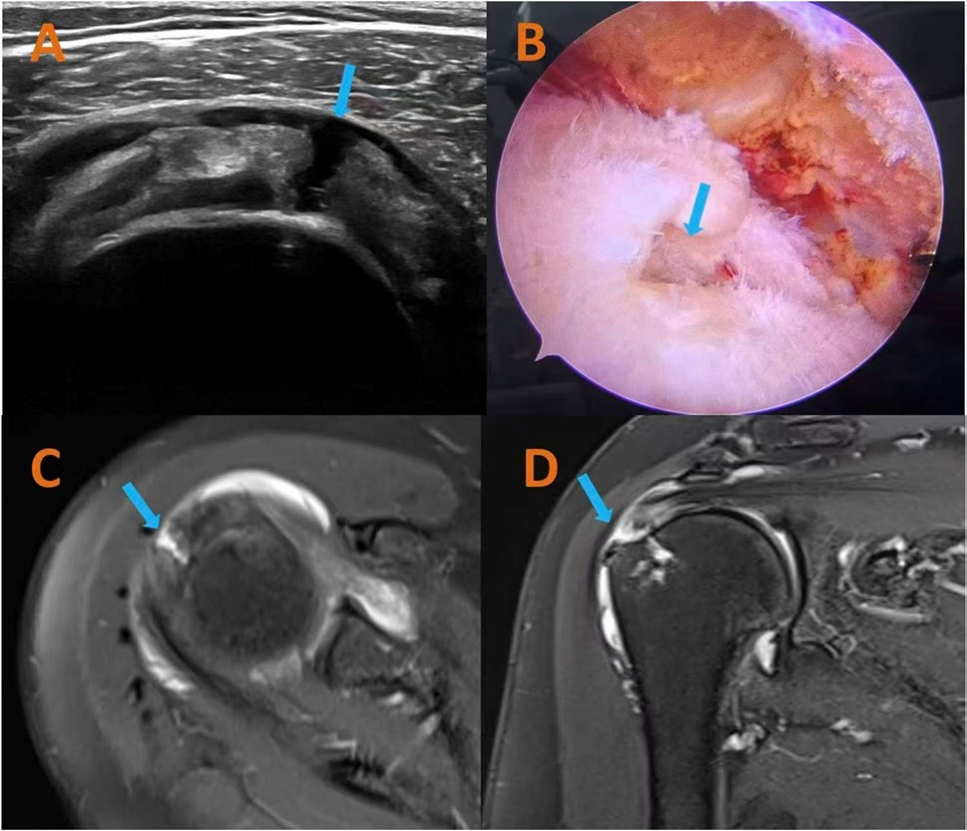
Ultrasound, magnetic resonance imaging (MRI) images, and intraoperative photographs of typical rotator cuff tendon tears. A Ultrasound image of a full-thickness tear of the rotator cuff. The arrow indicates the site of the tendon tear. B Intraoperative images of the patient during the arthroscopic surgery. The arrow indicates the site of the tendon tear. C Magnetic resonance transverse image of full-thickness tear of the rotator cuff. The arrow indicates the site of the tendon tear. D Magnetic resonance coronal image of full-thickness tear of the rotator cuff. The arrow indicates the site of the tendon tear
